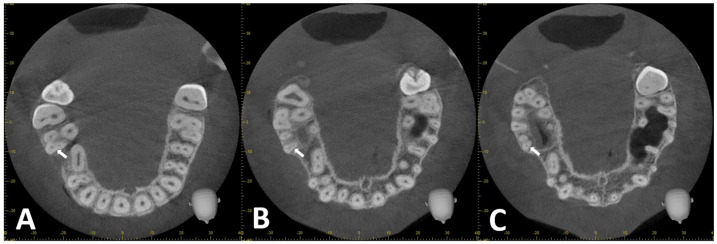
CBCT reconstructions of MB root in maxillary first molar tooth. (A–C) Coronal to apical axial sections exhibiting Vertucci type II RCC.
